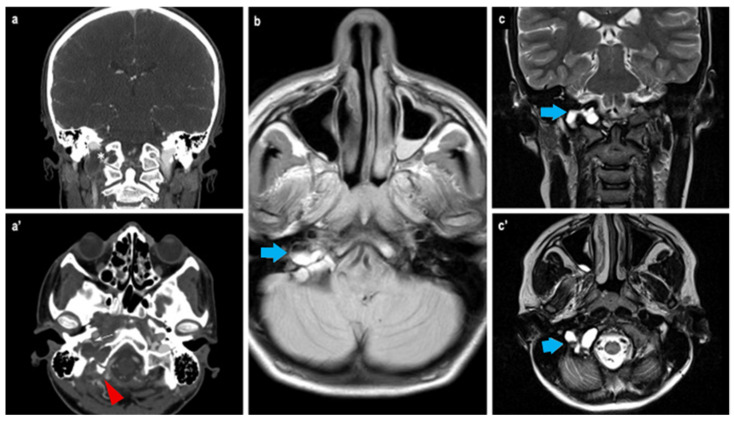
Radiological findings at the CT scan (a,a’): it is possible to appreciate the bony erosion caused by the extracranial expansive lesion at the right occipital condyle (white asterisk, red arrowhead) with direct contact to the sigmoid sinus. At MRI (b,c,c’), the presence of the characteristic fluid–fluid interface of aneurysmal cyst is clearly visible (blue arrows).
